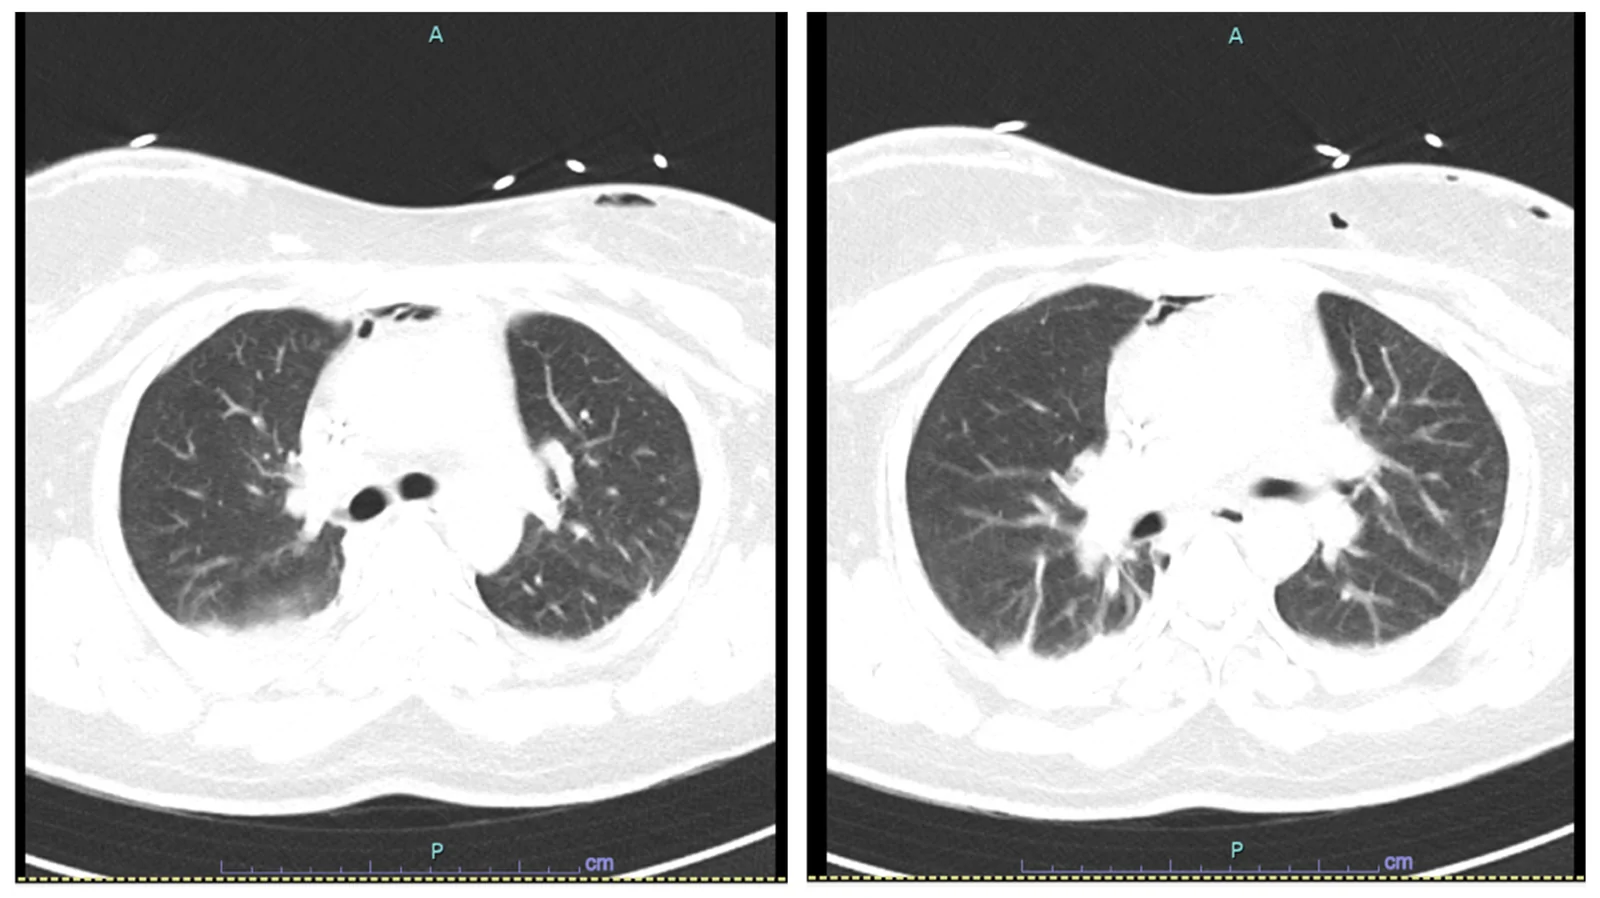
CT chest showing stable pneumomediastinum.
